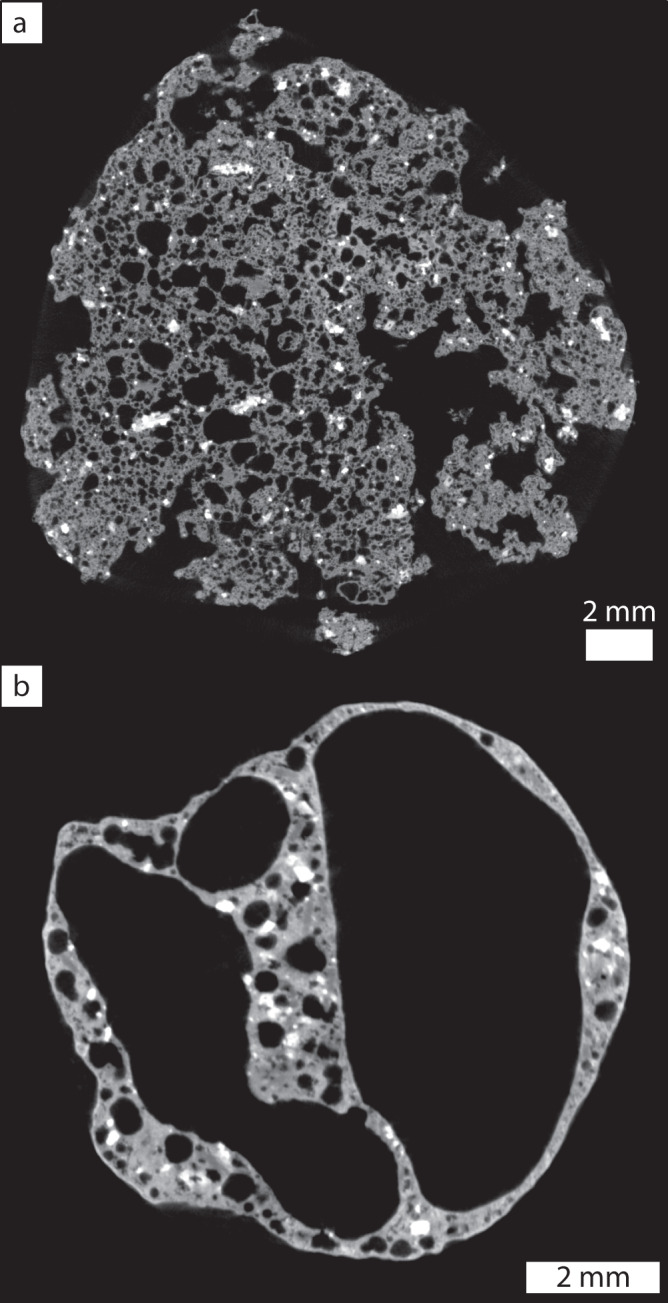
X-ray computed tomography derived images. a Image slice through regular scoria found adjacent to the inflated pyroclasts within the same deposit. This scoria clast has a bulk vesicularity of 76.5%. b Image slice through an inflated pyroclast. This inflated pyroclast has a bulk vesicularity of 89.7%. Note the difference in scale bar length between the two images.
